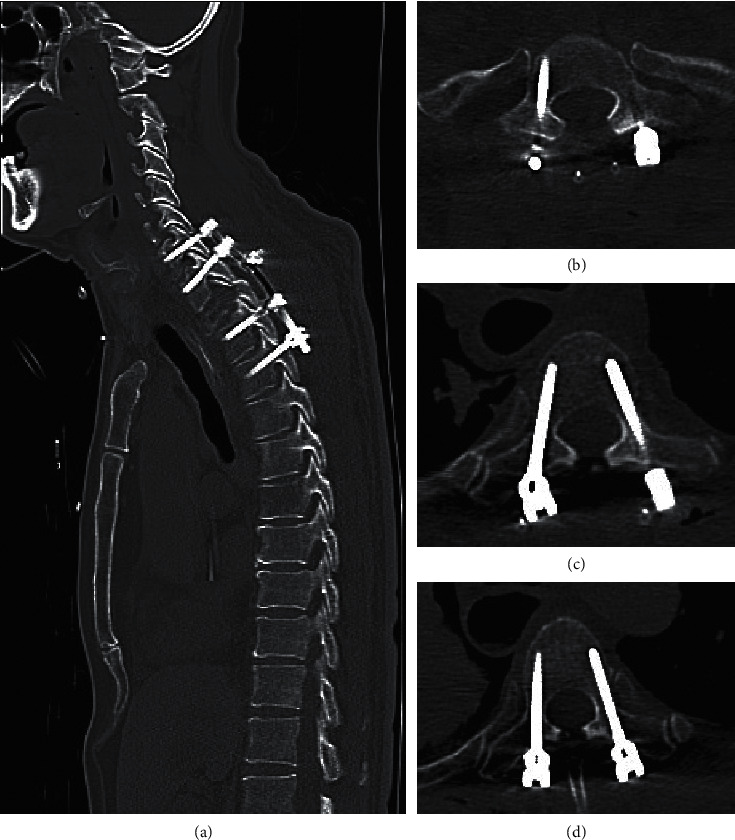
Postop immediate CT revealed all screws had good position: (a) sagittal view, (b) T1 level screws, (c) T3 screws, and (d) T4 screws.
